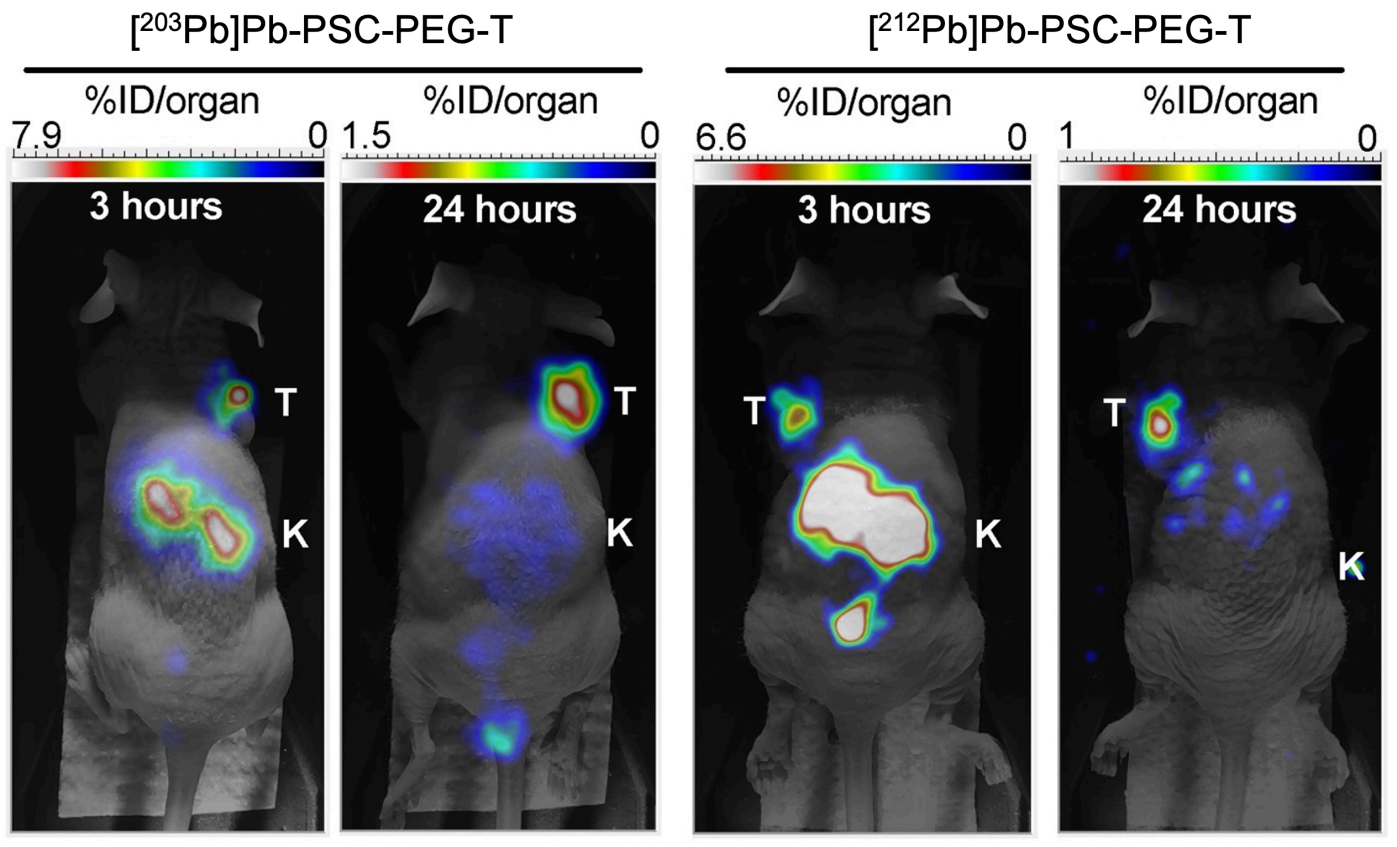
Preclinical comparison of ^203^Pb and ^212^Pb SPECT imaging, demonstrating the similar biodistribution and tumor-targeting properties of the ^203^Pb imaging and ^212^Pb therapeutic compounds. Adapted with permission from 123, copyright 2023 MDPI.
